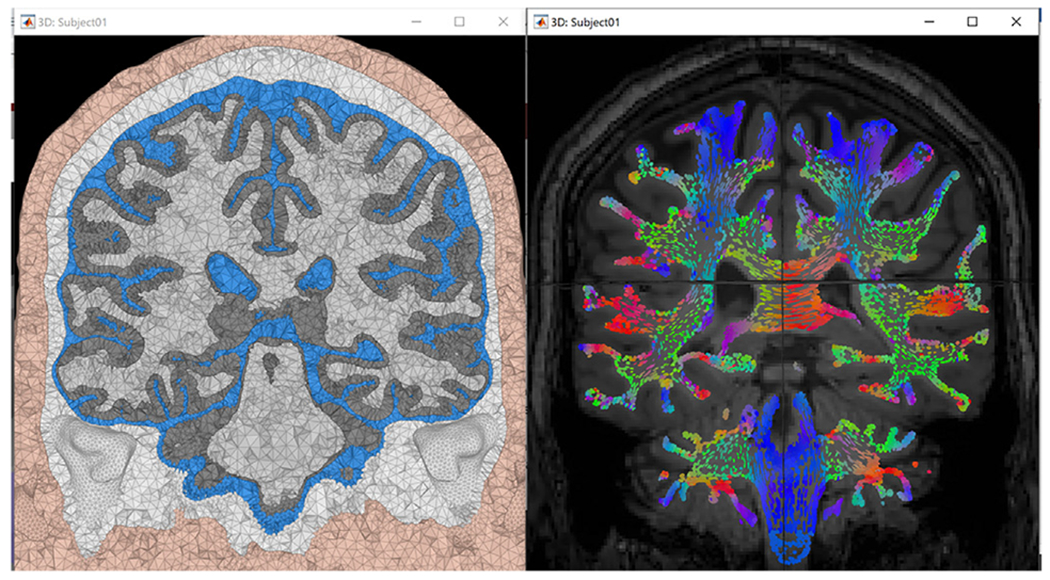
Visualization of the realistic FEM volume conductor with Brainstorm. Left: The tetrahedral volume mesh with five compartments: wm, gm, CSF, skull, and scalp. Right: The conductivity tensors in wm, overlaid on the subject’s MRI, as color-coded ellipsoids computed from the DWI data: red for dominant eigenvector oriented right-left, green for anterior-posterior, and blue for superior-inferior.
